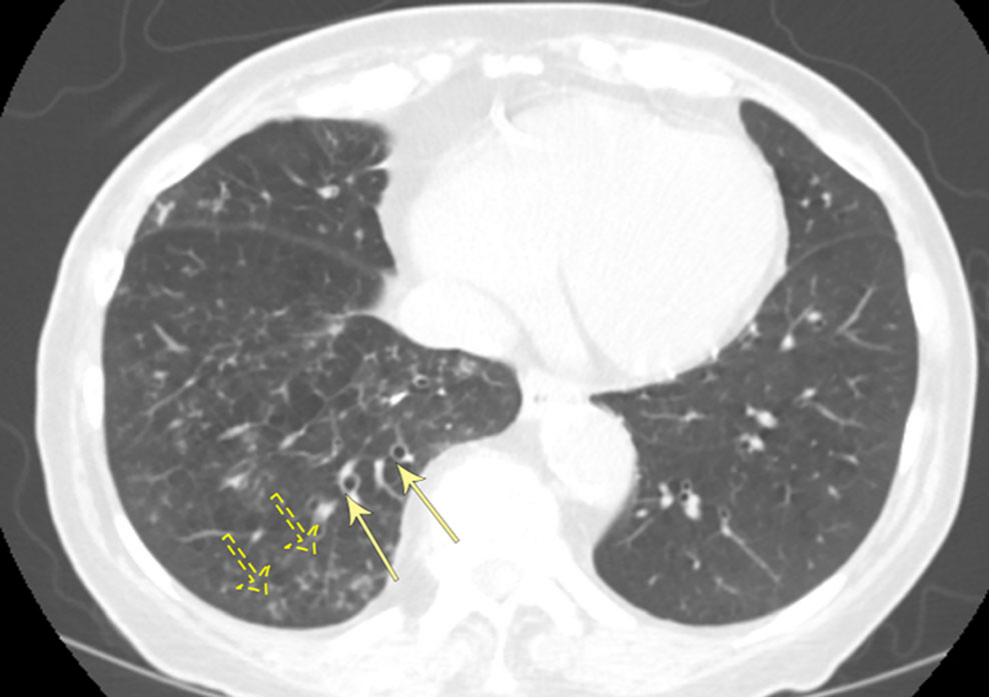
Bronchiectasis due to recurrent aspiration. Axial CT imaging demonstrates sequela of chronic/recurrent aspiration with lower lobe-predominant bronchiectasis (*solid arrows*) and multiple ill-defined centrilobular and tree-in-bud nodules in the dependent portion of the right lower lobe (*dashed arrows*)
